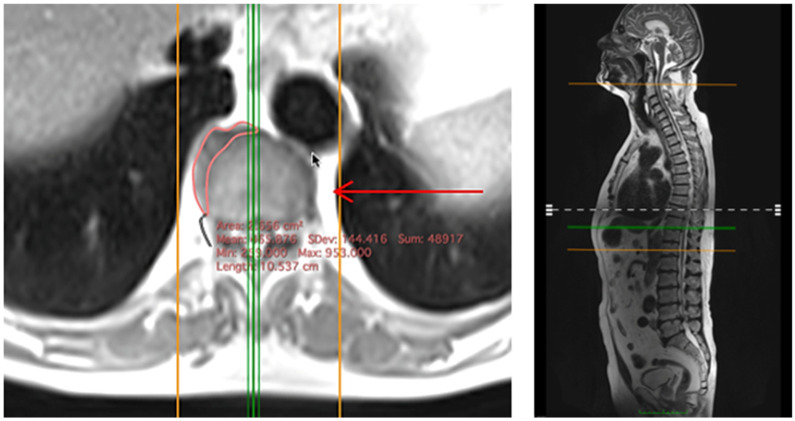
MRI-based measurement of thoracic spondylophytes. Spondylophyte size at T8–T11 was measured on axial T2-weighted MRI.
